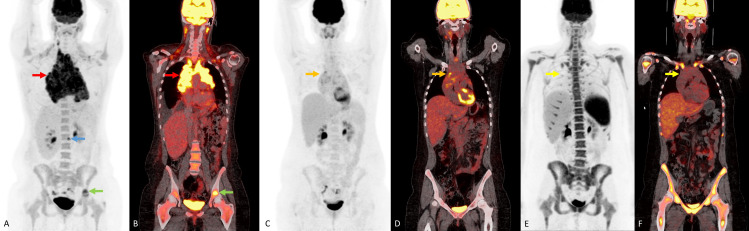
FDG-18 PET/CT MIP image (A) and coronal FDG PET/CT fusion images (B) for initial staging demonstrate FDG-avid bulky mediastinal and cervical nodes (red arrow). FDG-avid osseous lesions at the L2 vertebral body (blue arrow) and FDG-avid osseous lesion in left acetabulum (green arrow). Also note the physiologic brown fat uptake in the cervical and supraclavicular regions bilaterally. F-18-FDG PET/CT MIP (C) and coronal FDG PET/CT fusion image (D) for restaging after 2 cycles demonstrate decreased sized and FDG activity in the mediastinal and cervical nodes (orange arrow) consistent with a Deauville 4 response. F-18-FDG PET/CT MIP (E) and coronal FDG PET/CT fusion image (F) after chemotherapy escalation demonstrate a further decrease in FDG uptake in the mediastinal and cervical nodes (yellow arrow) consistent with a Deauville 2 response. Also note the diffuse increase uptake in bone marrow and spleen, likely secondary to chemotherapy. Abbreviations: FDG, fluorodeoxyglucose; MIP, maximum intensity projection; PET/CT, positron emission tomography–computed tomography.
